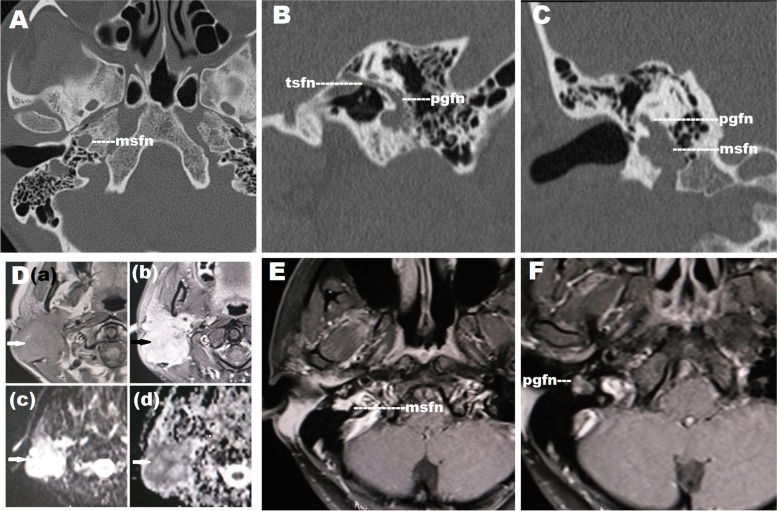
HRCT and CE-MRI findings of adenoid cystic tumor of right parotid (arrow) in a 26-year-old female patient showing perineural extension along the facial nerve. A HRCT scan in axial section showing the enlarged canal for mastoid segment of facial nerve (msfn). B HRCT in oblique sagittal section showing enlarged posterior genu of facial nerve (pgfn) with 3 mm extension into tympanic segments (tsfn) of facial nerve. C HRCT in oblique coronal section showing enlarged posterior genu (pgfn) and mastoid segments (msfn). D (a, b, c, d). (a) Showing the parotid lesion as isointense on T1WI, (b) enhancing on post contrast T1WI, (c) hyperintense on DWI, and (d) hypointense on ADC maps indicating restriction of diffusion. E Axial contrast enhanced MRI showing enhancement of mastoid segment of facial nerve (msfn). F Axial contrast enhanced MRI showing patchy enhancement with thickening of the posterior genu of facial nerve(pgfn)
